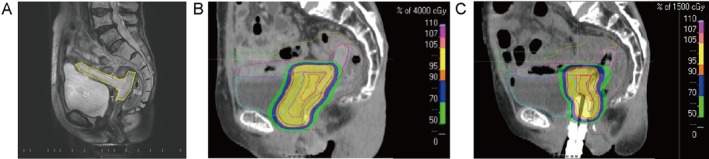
Treatment planning after bioabsorbable spacer placement. (A) MRI (sagittal T2‐weighted image) showed that the bioabsorbable spacer (yellow dotted line) had created distance between the vaginal recurrent tumor (T) and rectum (Ra). The distance created by the spacer insertion between the tumor and the rectum was 13 mm. Bl indicates the bladder. (B, C) Dose distribution in radiation therapy planning. (B) Dose distribution up to 40 Gy, (C) Dose distribution after 40 Gy.
